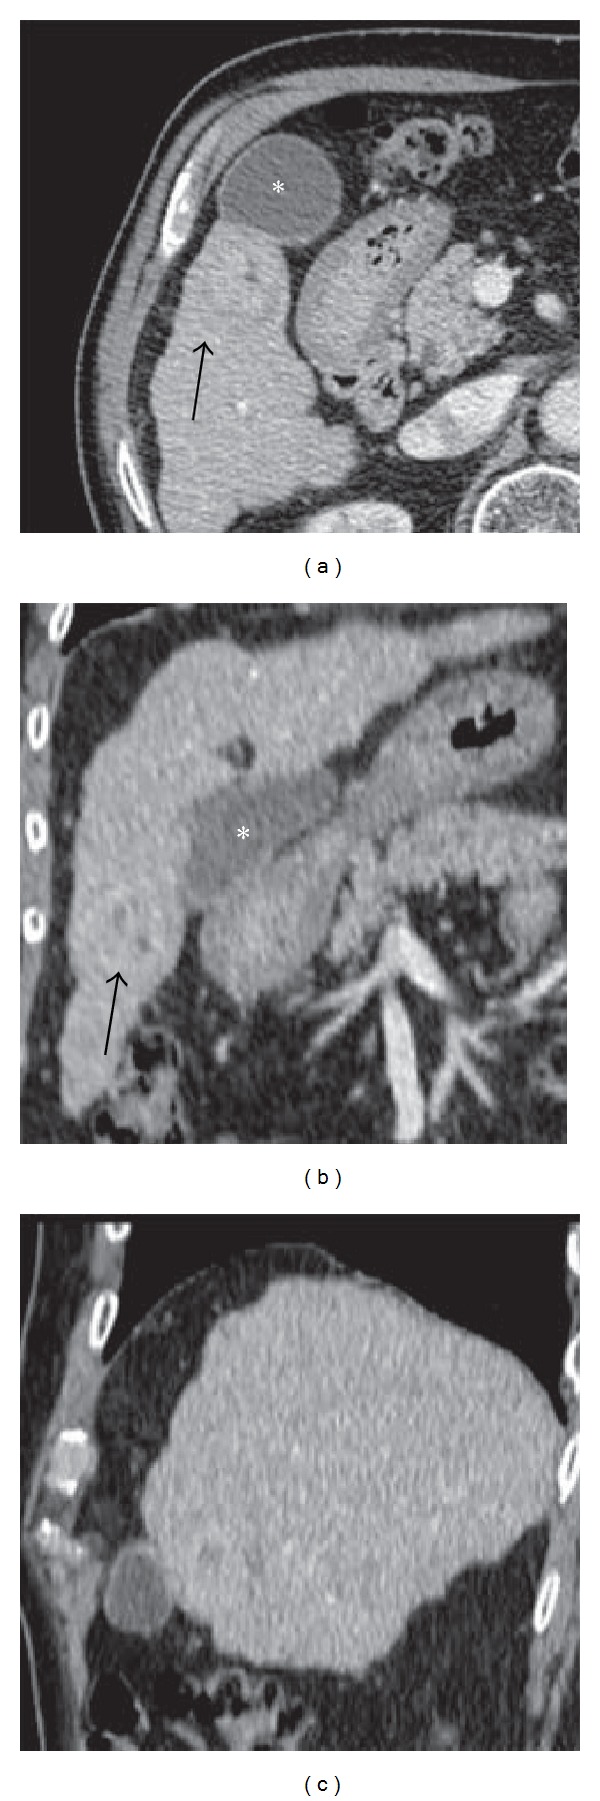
CT Preprocedural axial image (a) and multiplanar reconstructions (b, c) show the relationship between hepatocellular carcinoma (black arrow) and the gallbladder (∗).
